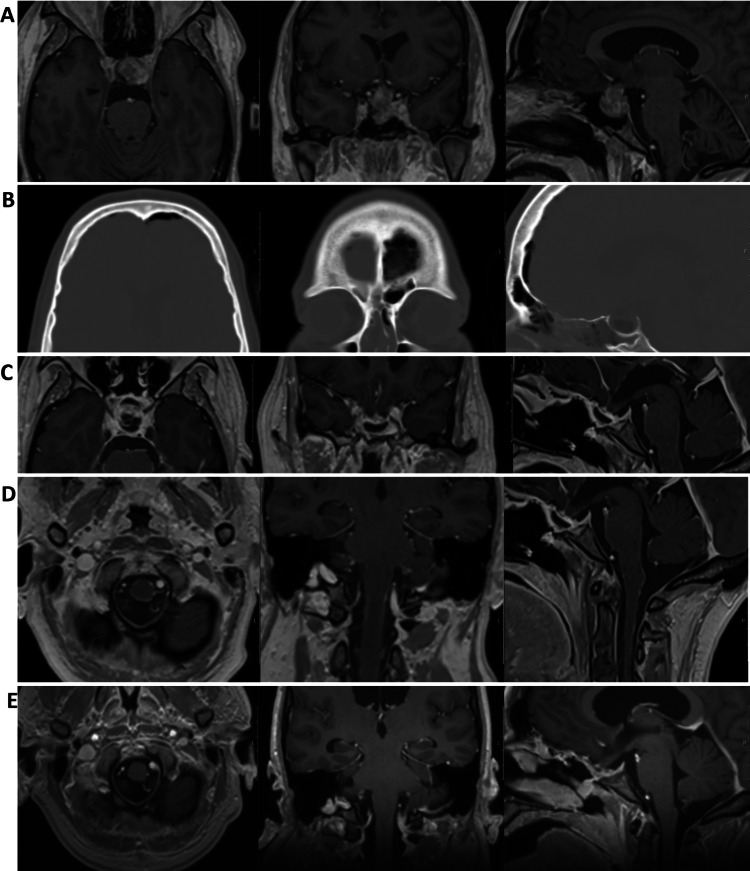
A) CE T1-w axial, coronal, and sagittal MRI brain images. A 2.5 cm large suspected macroadenoma engaging the hypophyseal stalk with compression of the optic chiasm and parasellar extension. B) Axial, coronal, and sagittal CT brain, bone window. Post-resection status, small amount of pneumocephalus. Fat grafting has been performed at the tumor site and within the sphenoidal sinus (sagittal view). C, D) CE T1-w axial, coronal, and sagittal MRI brain images. Signs of extensive leptomeningitis in the sellar and infratentorial (C) and in the infratentorial and spinal region (D). Appearance consistent with adhesions in the sella, deformed suprasellar structures, and edema in the markedly displaced optic chiasm. No definitive signs of cerebrospinal fluid leakage were observed. E) CE T1-w axial, coronal, and sagittal MRI brain images. Partial regression of leptomeningeal contrast-enhancement in the infratentorial and spinal region. Unchanged postoperative status in the sellar region with displacement of the optic chiasm
